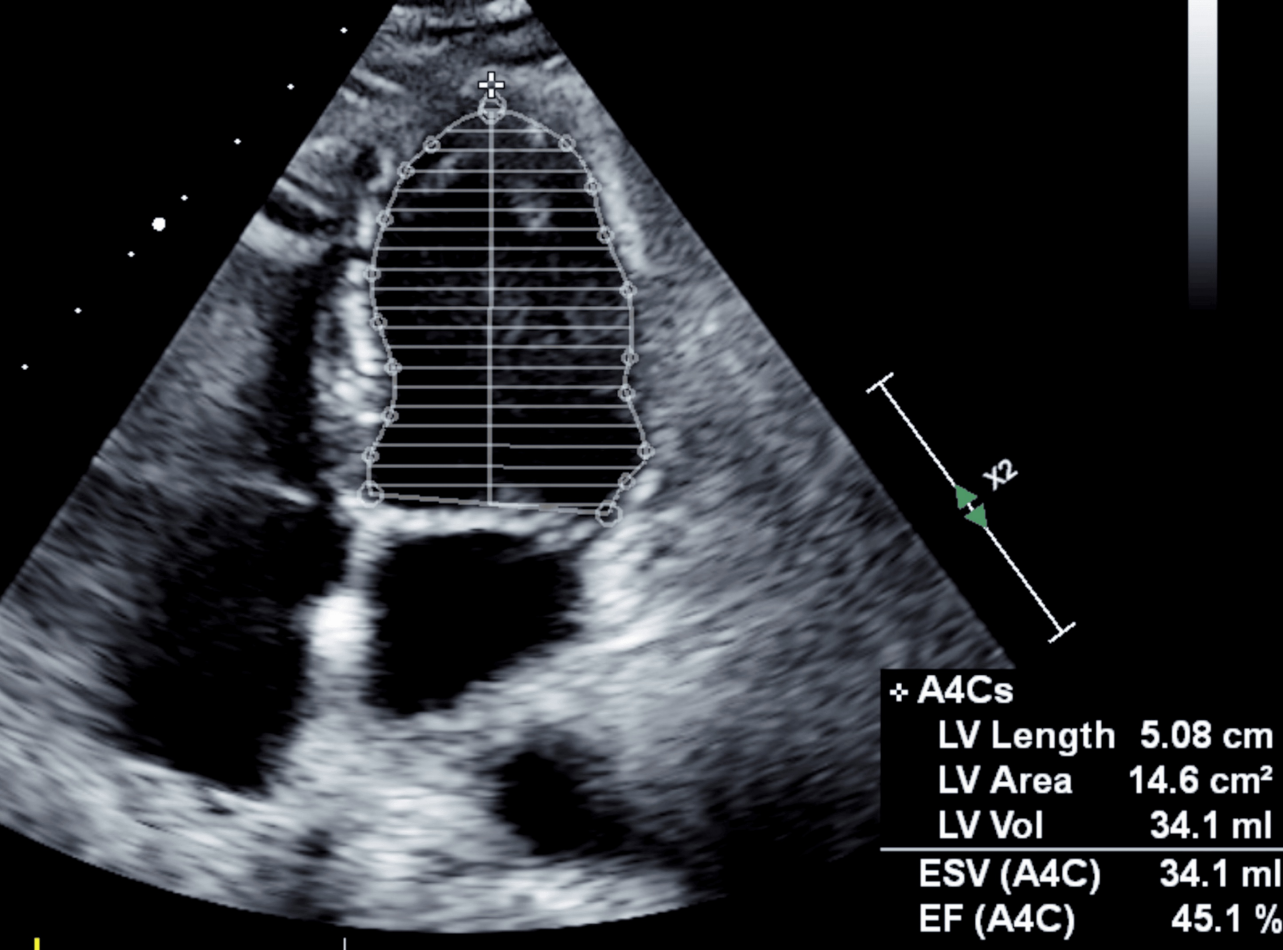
Echocardiogram in the apical four-chamber view, showing an ejection fraction of 45% A4C: apical four-chamber view; LV: left ventricle; ESV: end-systolic volume; EF: ejection fraction
